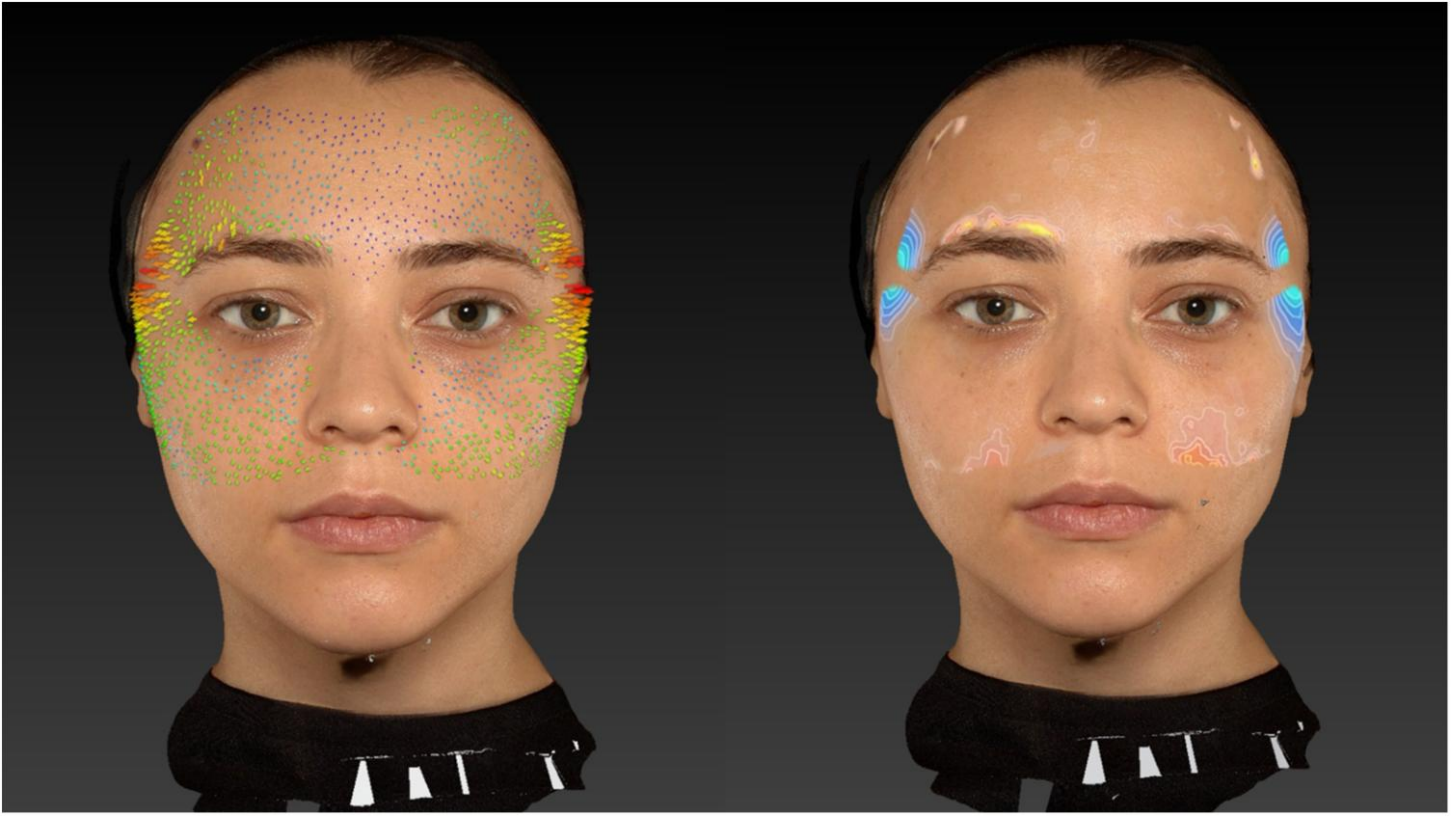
Frontal view before and after interfascial filler placement in the bilateral temporal regions. The image demonstrates restoration of lateral facial fullness, smoothing of temporal depressions, and a lifting effect on the upper third of the face, most notably in the temporal and lateral brow areas. Volumization of the temporal hollowing contributes to a more youthful, balanced facial contour. Key reference points include the supraorbital rim, lateral brow, and anterior temporal line, used to assess post-treatment symmetry and vertical vector lifting. The filler placement was confirmed sonographically within the interfascial compartment.
